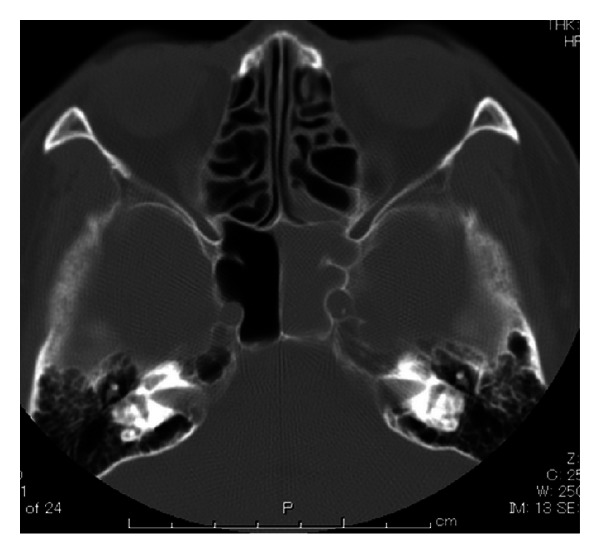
CT findings. The left sphenoid sinus shows a soft tissue shadow without bone damage.
